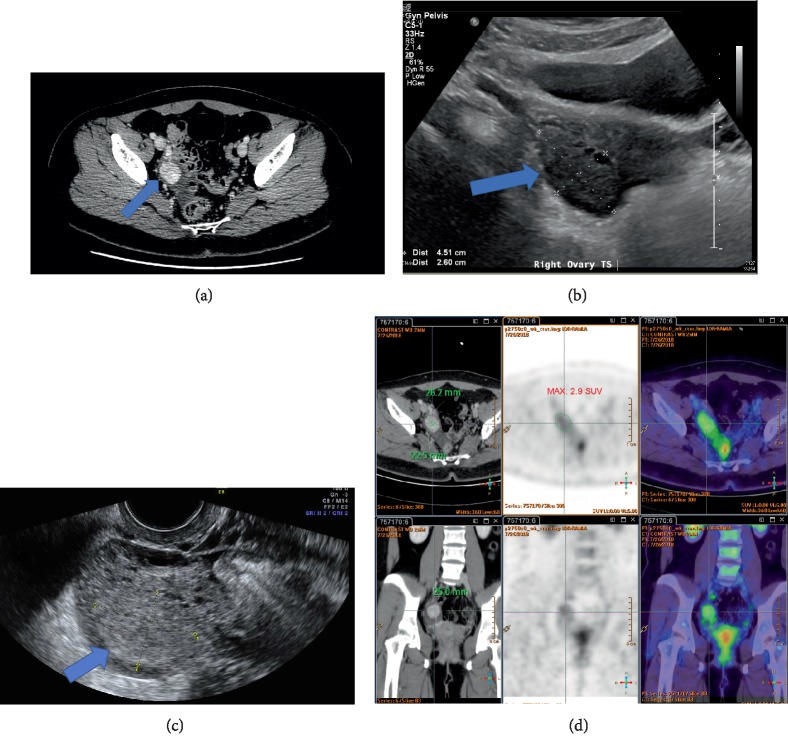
(a) Contrast CT scan of the abdomen and pelvis showed an enlarged 2.6 × 2.3 × 2.5 cm right ovary (blue arrow). (b) Transabdominal ultrasound showed an enlarged 4.5 × 2.6 × 4.5 cm right ovary five months after CT scan (blue arrow) (c) Transvaginal ultrasound showed a 2.6 × 2.4 × 2.2 cm hyperechoic solid tumor inside the right ovary (blue arrow) (d) FDG PET–CT scan with contrast showed a 2.6 × 2.3 × 2.5 cm mildly FDG-avid mass (SUV_max_ 2.9 g/ml) in the right ovary (tumor dimensions labelled).
